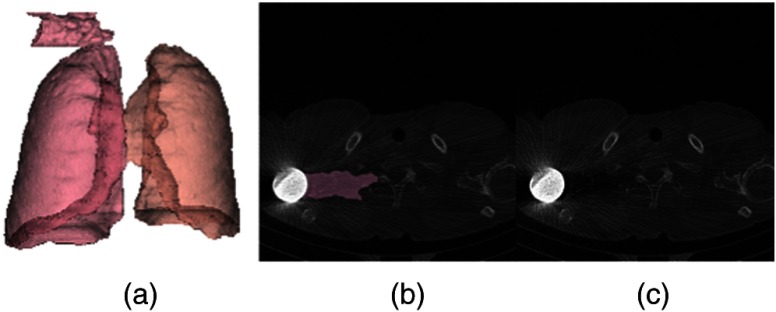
Example unacceptable lung segmentation (a) segmentation shown in 3-D, (b) segmentation shown in 2-D, and (c) intensity CT image of the same axial slice.
